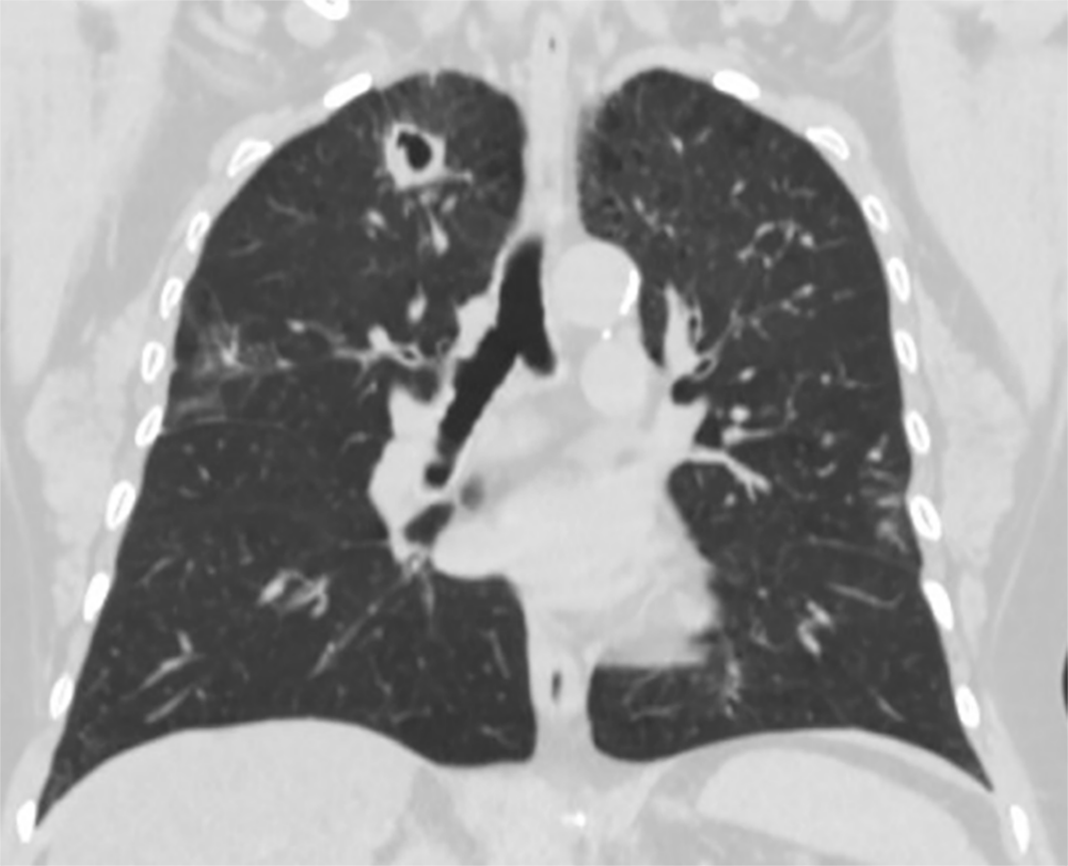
A 62-year-old woman with a history of COPD, asthma, and type 2 diabetes presented with chronic dyspnoea. A chest CT revealed a pulmonary cavitation in the right upper lobe. Bronchoalveolar lavage culture grew *Histoplasma capsulatum*.
